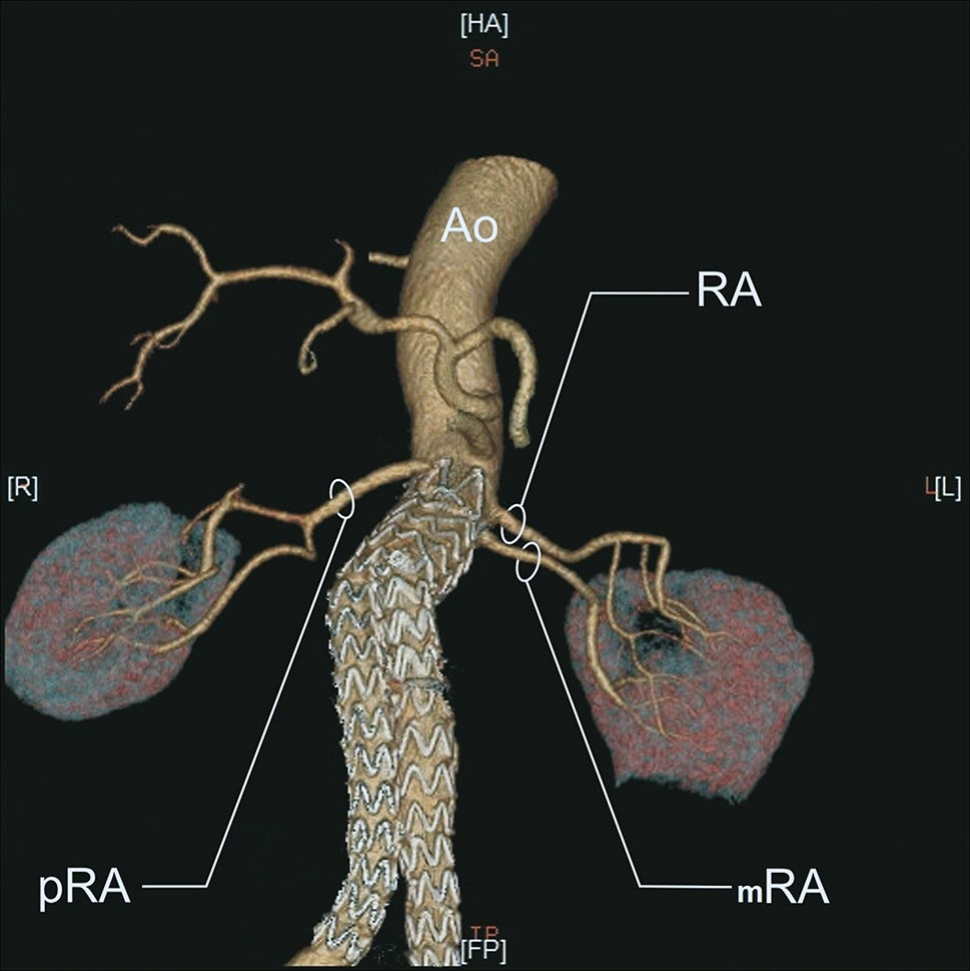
Three-dimensional computed tomographic angiography reconstruction of the abdominal arteries. *Ao* abdominal aorta, *RA* renal artery, *pRA* prehilar renal artery, *mRA* multiple renal artery
